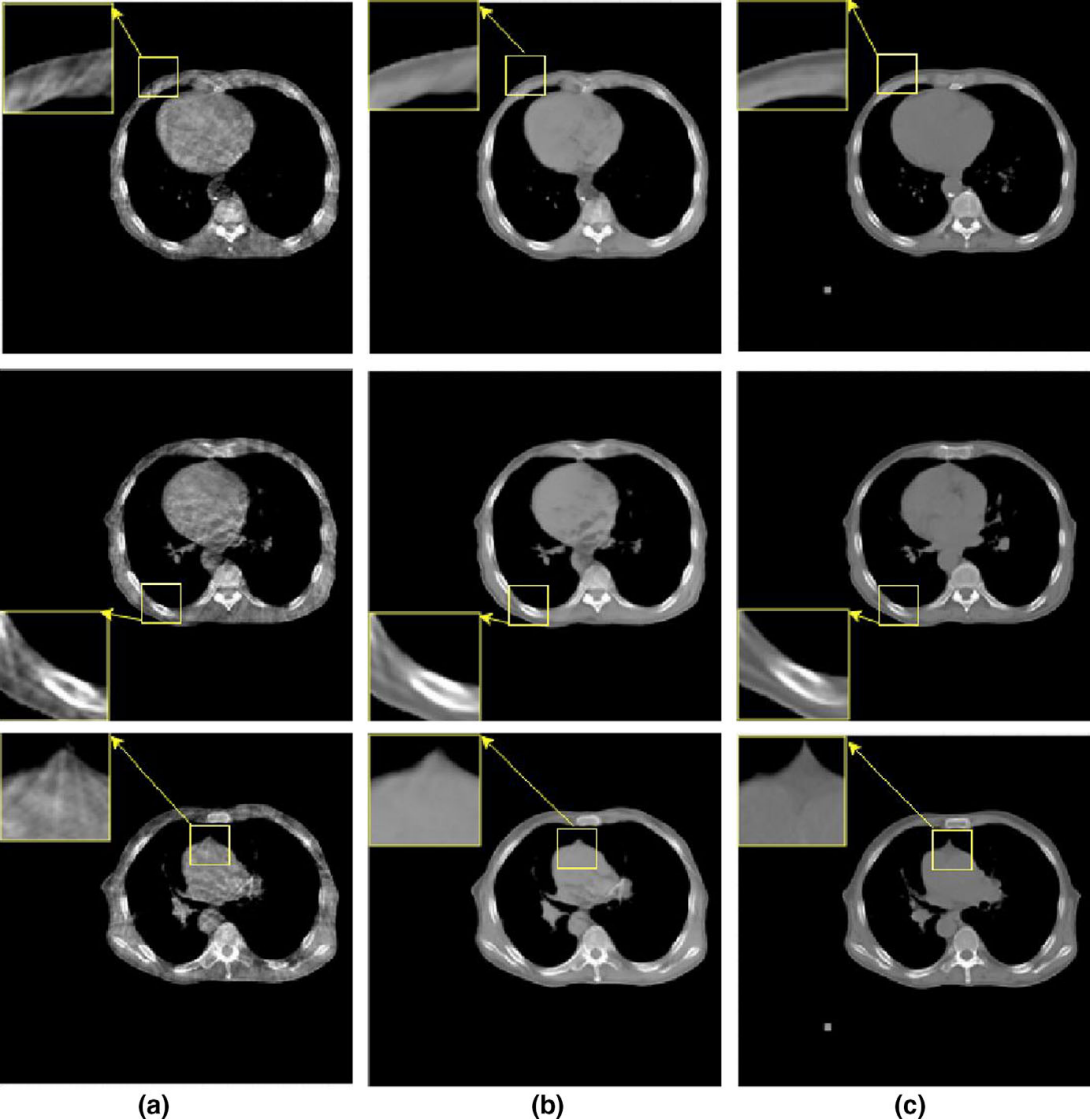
Artifact removal results obtained with the chest data. (a) CBCT, (b) proposed correction, and (c) RCT. Display window [−401, 526].
